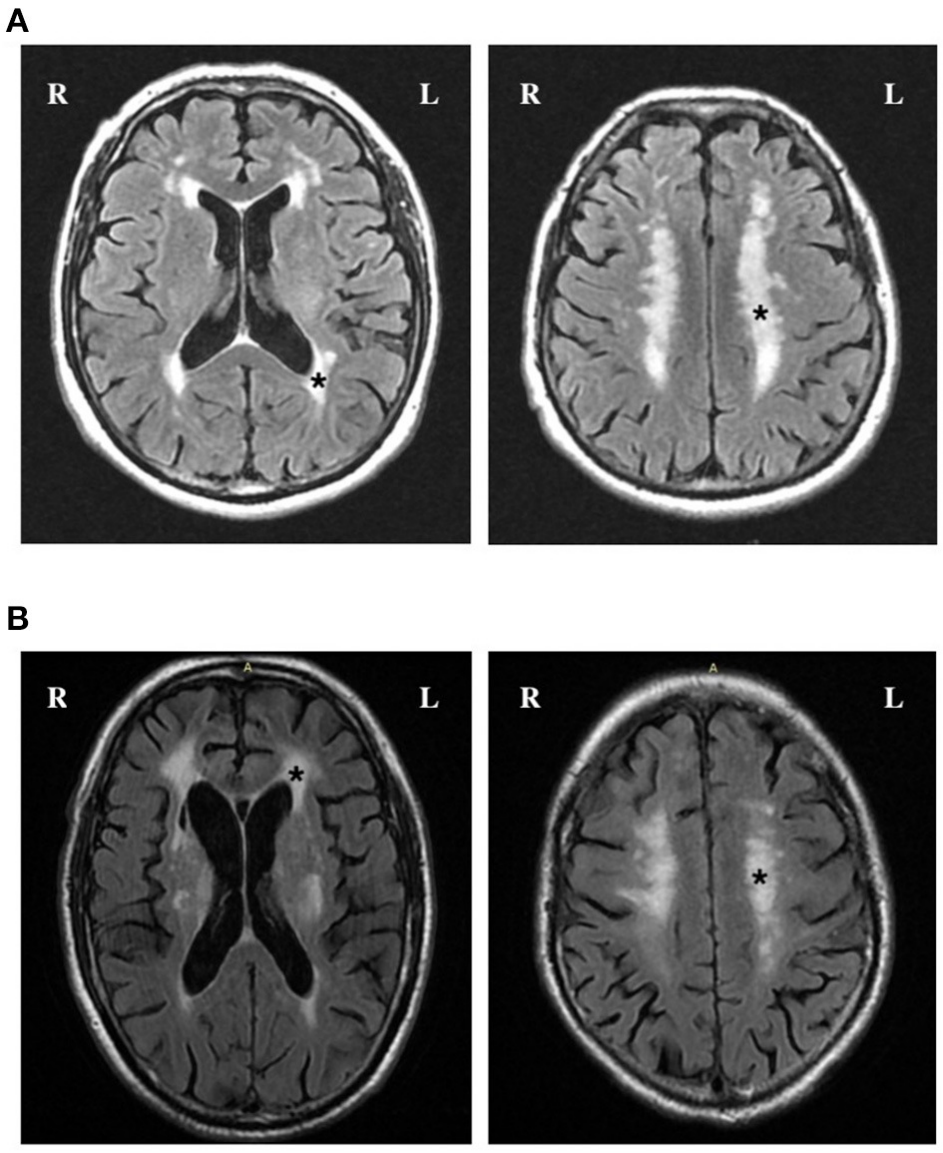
FLAIR images of high total WMH burden in patients with elevated TMAO levels. (A) and (B) Axial FLAIR slices show the position of periventricular (left) and deep WMH (right). Fazekas scores in periventricular region and deep region were both rated as grade 3 (^*^) in a patient with 5.3 μmol/L TMAO (A) and 5.4 μmol/L TMAO (B).
